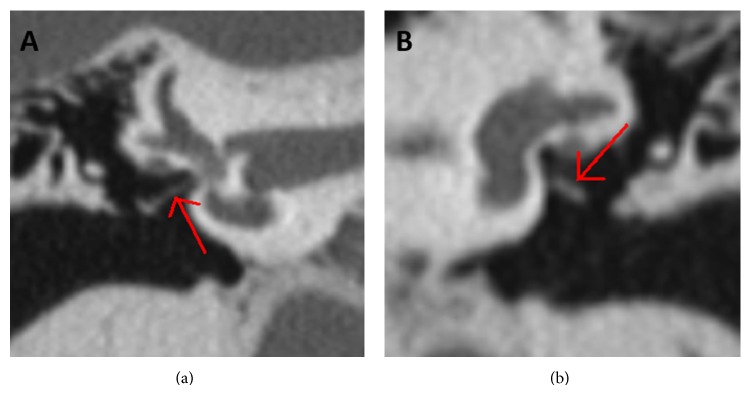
(a) Coronal section of CT showing normal position of stapes and tympanic portion of facial nerve on the right ear. (b) Coronal section of CT showing dislocation of stapes suprastructure from footplate on the left ear.
